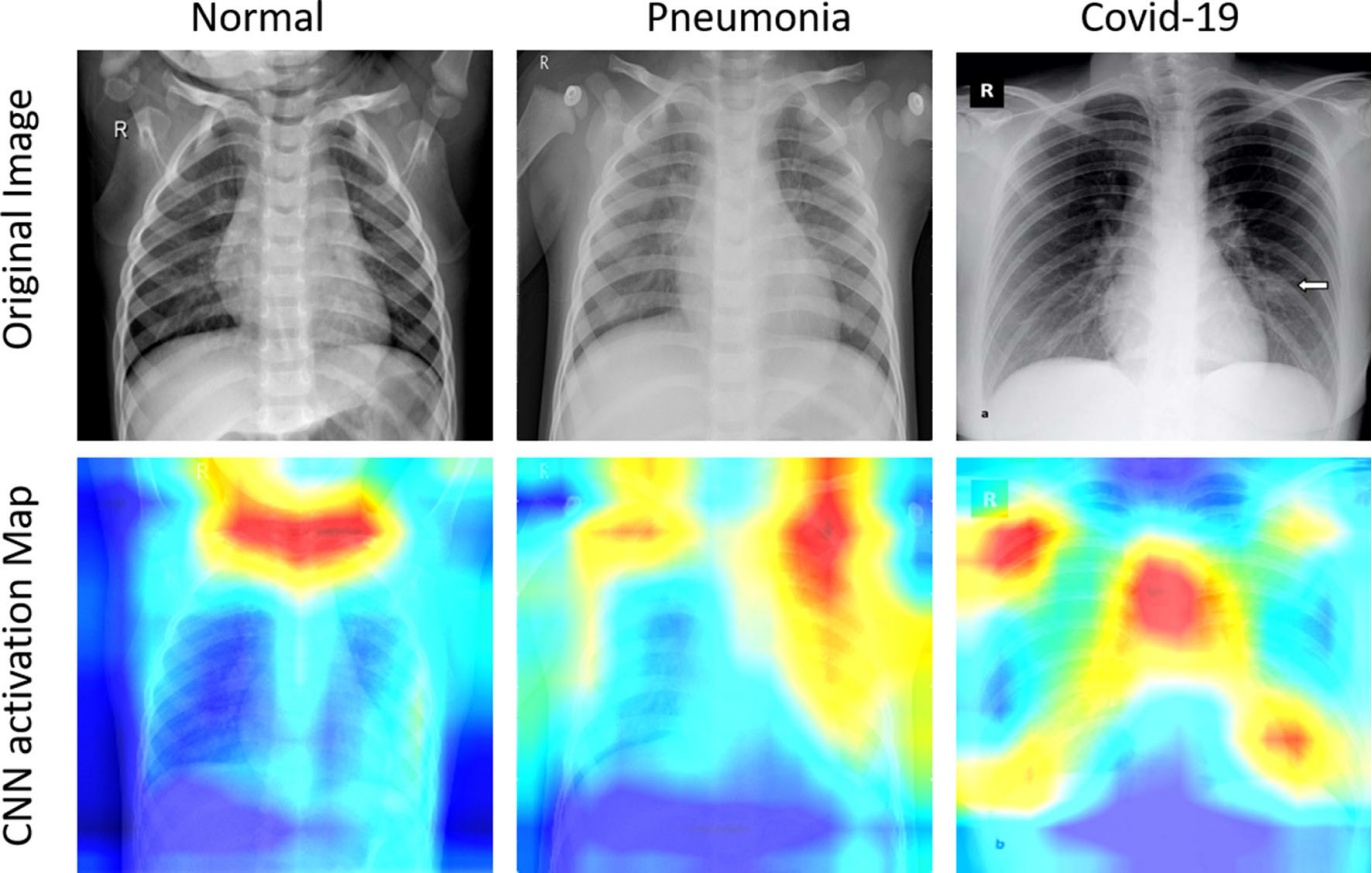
Activation map visualization for the three classes under consideration. The First column presents a healthy chest X-ray sample, the second, from a patient with Pneumonia, and the third one, from a patient with COVID-19, visualizing highly affected regions in red
